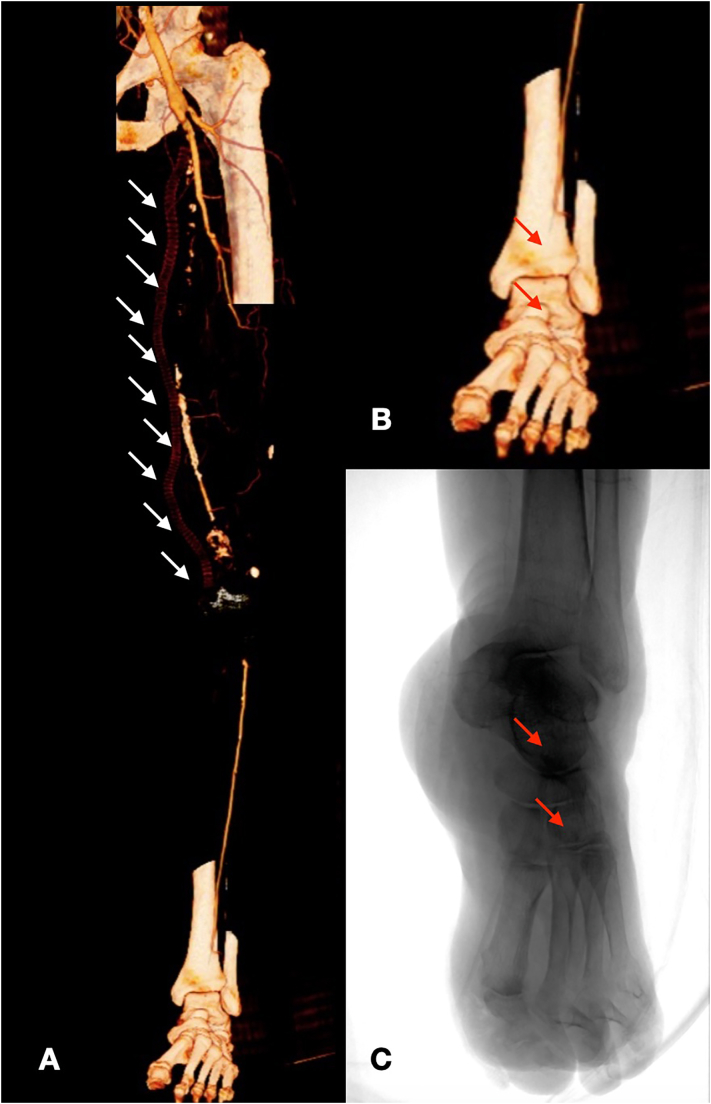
CT Angiography 3-dimensional volume rendering preoperative showing results of previous procedure (A) with the detail and intraoperative angiography of foot showing occlusion in ATA and the lack of pedidia artery (B and C).
